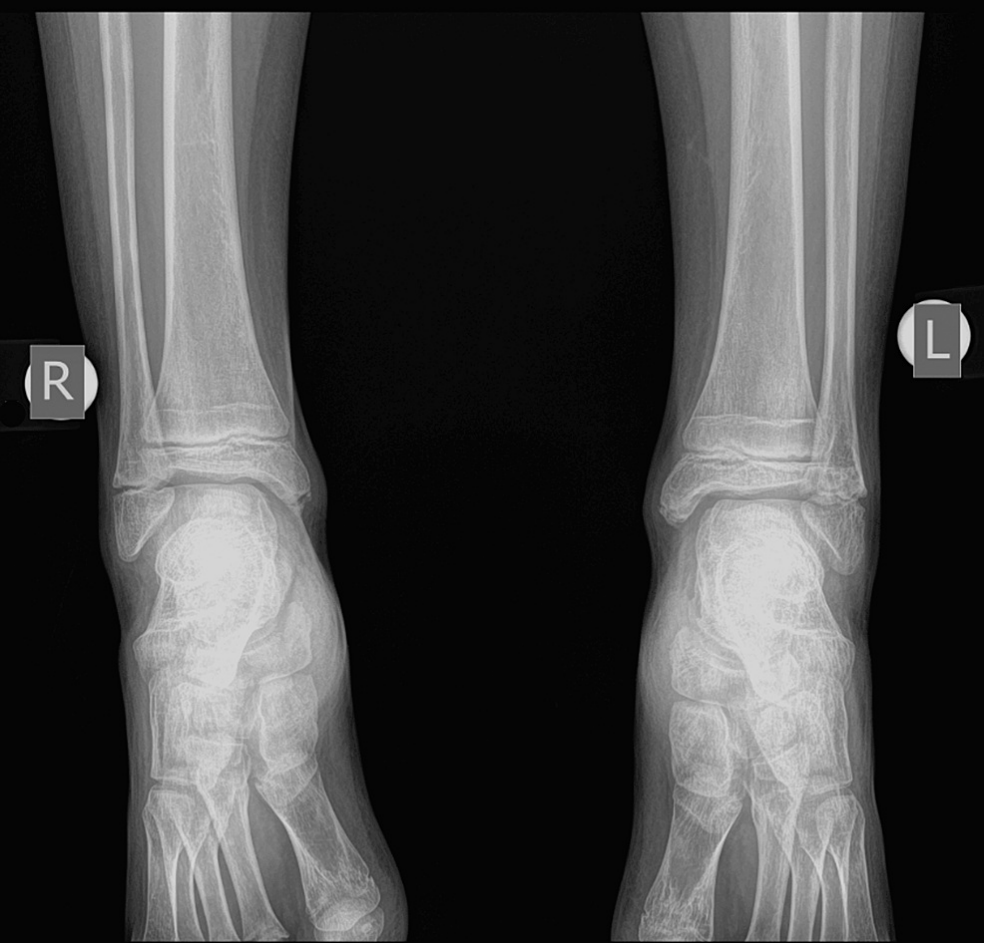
A comparative X-ray of both ankle joints, one year after injury, with normal growth of the left distal fibula.
